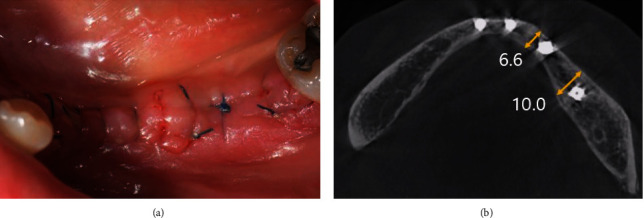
Postoperative intraoral (a) and radiograph views (b) (Case 2). The width of the alveolar bone was well expanded, and we can see that the implant had been placed in the right direction.
